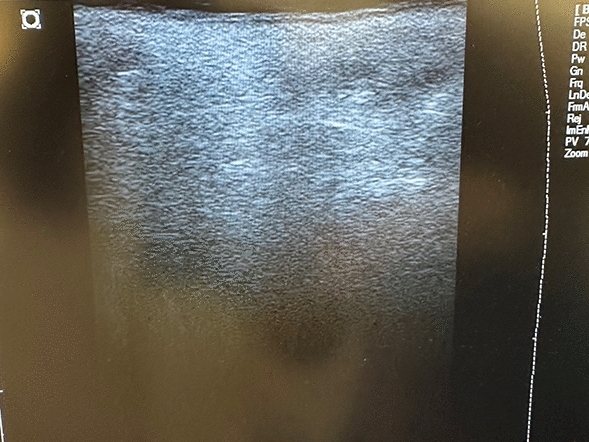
Ultrasonography of the gluteal area of a woman with a follow-up of 38 months from the PMMA removal. The tissues appear homogeneous. No PMMA residuals or fibrosis are present
